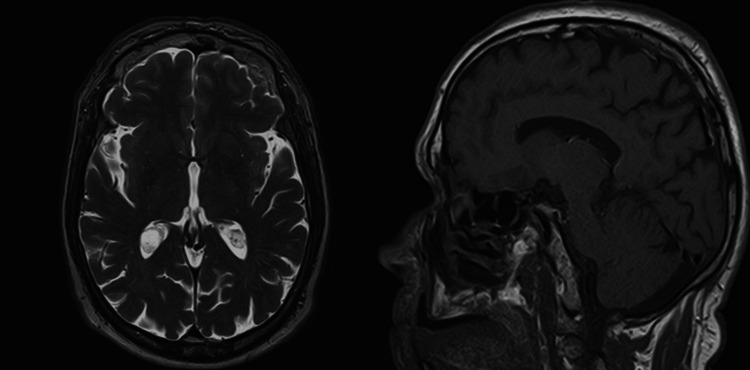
Brain MRI showing no metastasis
